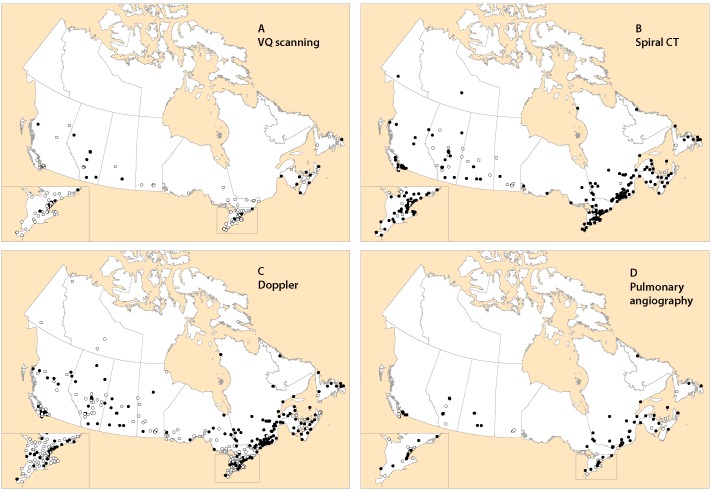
Availability of hospital-based diagnostic imaging services across Canada. Clear dots = limited services. Solid dots = service present 24/7. CT = computed tomography, VQ = ventilation–perfusion, 24/7 = 24 hours a day, 7 days a week.
